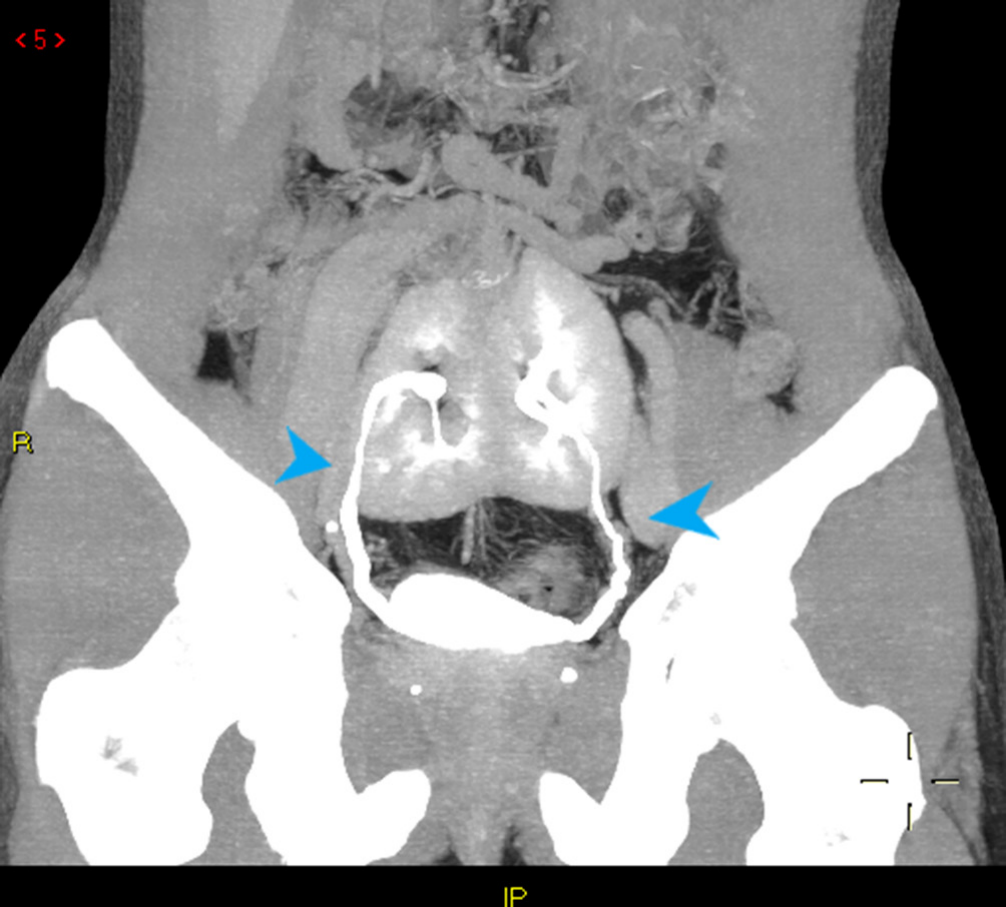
CT MIP coronal reconstruction of urinary tract anatomy: two ureters entering
